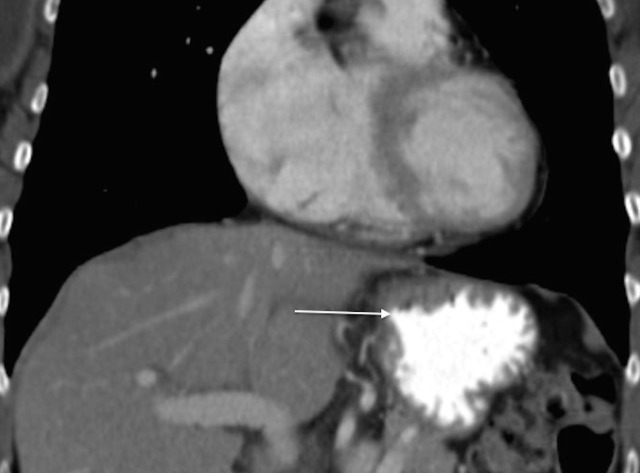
Contrast-enhanced CT image of the abdomen from Patient 2 after continued treatment with capecitabine monotherapy. This image shows continued absence of disease, with no appreciable mass in the gastric cardia.
